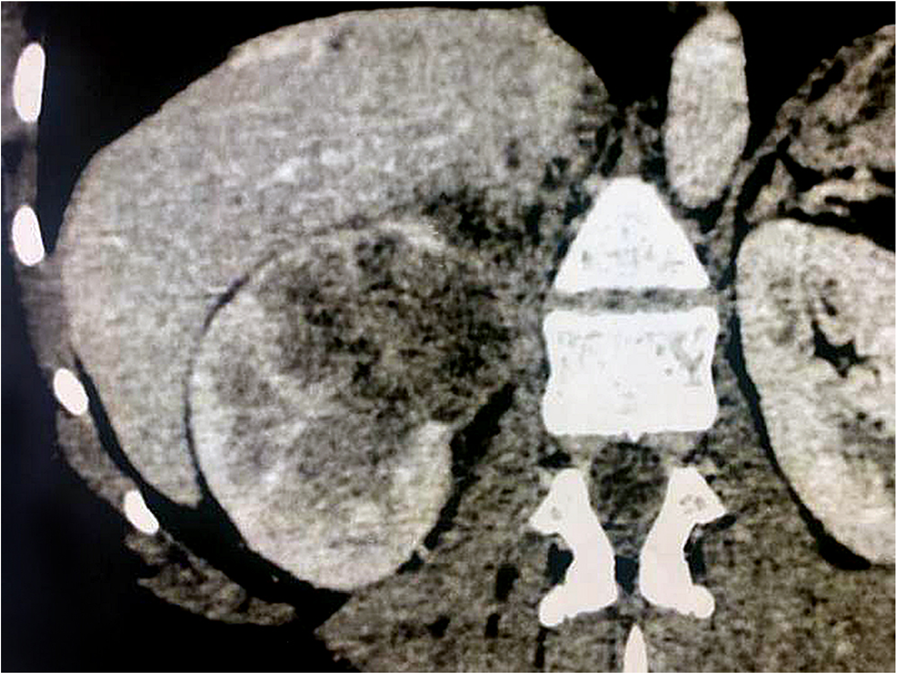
Computed tomography shows heterogeneous enhancing mass involving the upper and middle poles of the right kidney measuring approximately 8 × 7 cm
